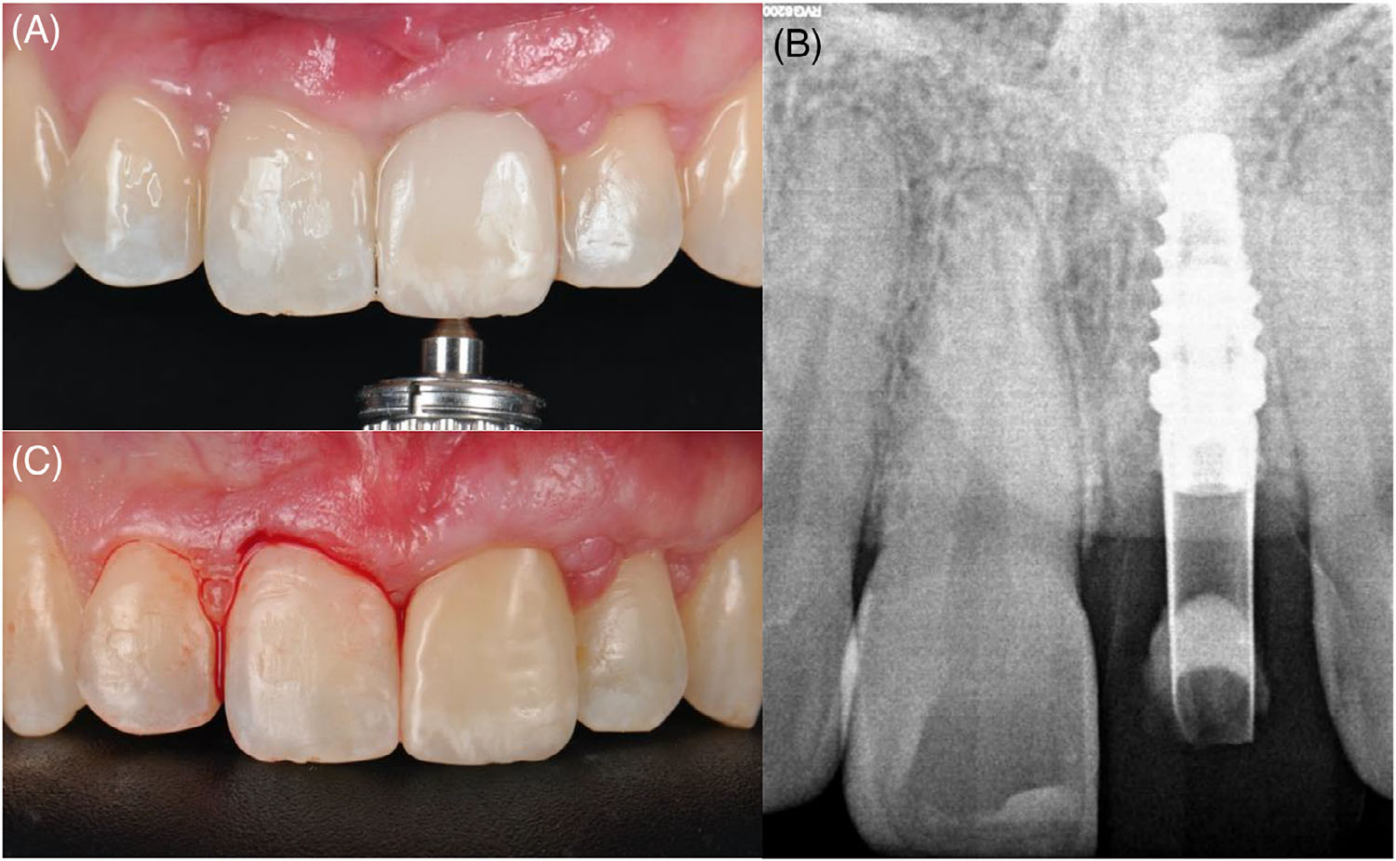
Implant loading. Frontal view, 2 weeks after provisional connection (A). Six weeks after provisional—some gingivectomy in the upper right central (C). Periapical radiograph (B).
